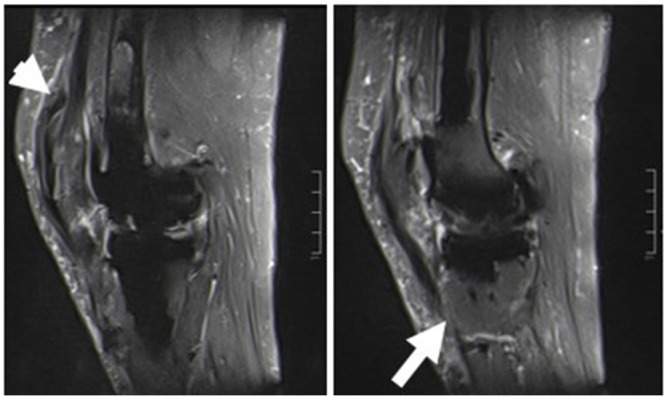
Long‐axis MRI scan performed 7 years after surgery. The graft extending from anteromedial aspect of quadriceps tendon in the distal portion of the thigh extending over the patellar tendon and reaching distally up to the tibial tuberosity is marked by arrows. MRI, magnetic resonance imaging.
